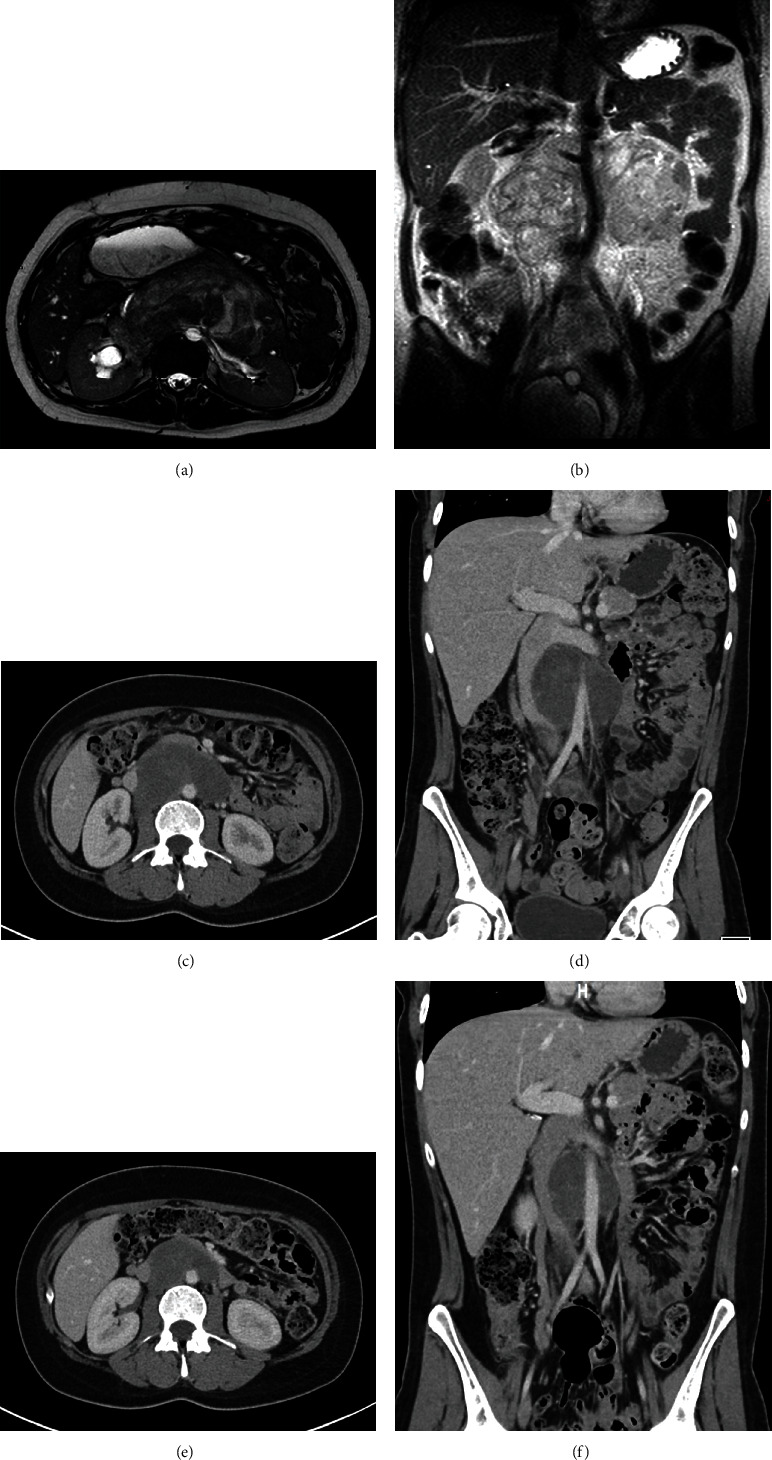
Radiology of synovial sarcoma case. Magnetic resonance imaging prior to treatment (a, b) demonstrating the large retroperitoneal mass. (a) Fluid sensitive axial image shows the mass partially encasing the abdominal aorta and compressing and displacing the inferior vena cava. Mild right hydronephrosis is evident. (b) T2 coronal image showing extensive encasement of the aorta and right renal artery by the large retroperitoneal mass. Computed tomography imaging post 3 cycles (c, d) and 6 cycles (e, f) of neoadjuvant chemotherapy demonstrating treatment response as evidenced by marked decrease in tumor size, decreased mass effect on the vena cava, resolution of right hydronephrosis, and deceased tumor density (suggestive of necrosis).
